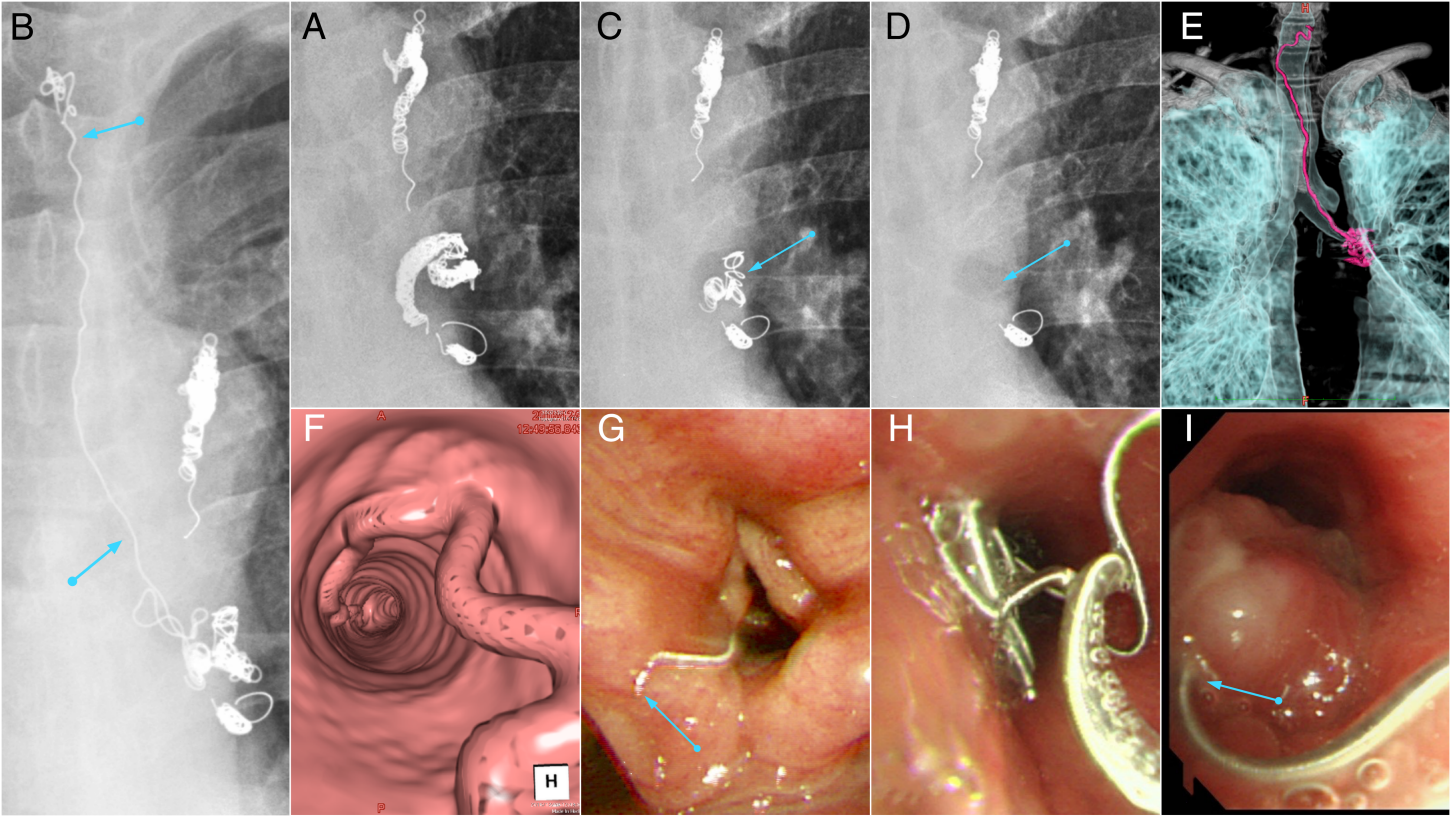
Case 2: (A) Chest radiography results immediately after the first bronchial artery embolization. The left upper and lower bronchial arteries were embolized. (B) Chest radiographs of erratic coil migration. A part of the coils placed in the left bronchial artery was observed in the left main bronchus and the trachea. Blue arrows indicate migrated coils in the trachea and the left main bronchus. (C) We retrieved the migrated coil fragment using a loop cutter and forceps. Blue arrow indicates the residual coils in the bronchial artery. (D) Three months later, the patient revisited us, reporting that she had coughed up a coil fragment. The residual coils observed in (C) disappeared (blue arrow). (E, F) Computed tomography volume‐rendering images of erratic coil migration. Migrated coils are coloured red in (E). (G–I) Bronchoscopy images of the migrated coil. The end of the coil (blue arrow) was moving in and out of the vocal cord (G). This was presumed to be the cause of severe dry cough. In (I) the blue arrow indicates the fistula through which the coil was protruding.
